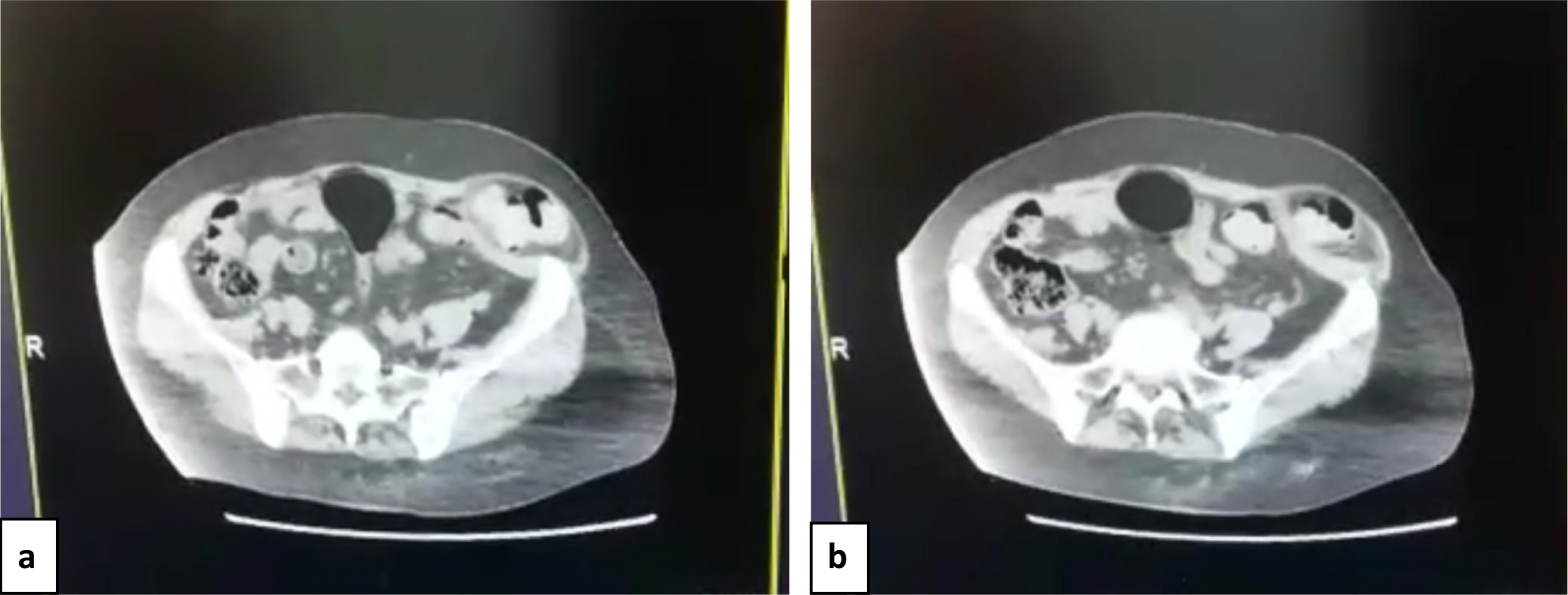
The CT‐Scan revealed a left inguinal hernia measuring 8.5 mm, containing loops of the small and large intestine, the end of the descending colon, and sigmoid colon, with suspicion of a sigmoid volvulus.
